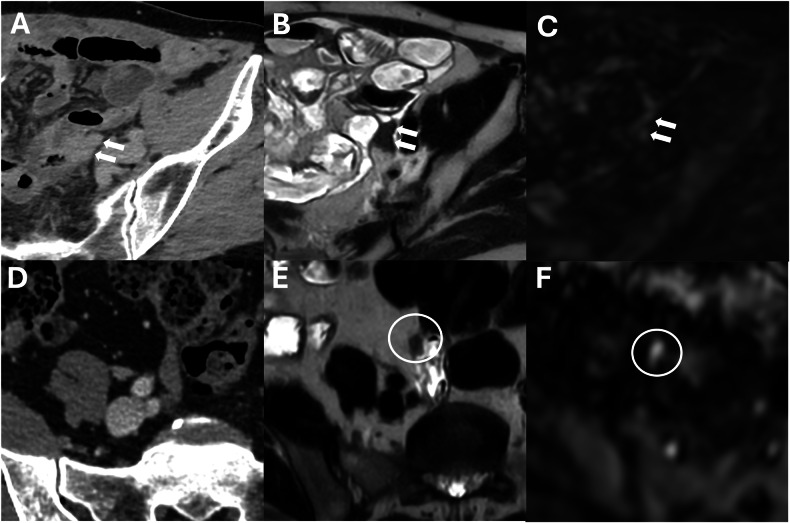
Ovarian cancer with peritoneal carcinomatosis in a 69-year-old woman (A–C). A CT delayed phase, axial plane showed a nodular implant in the left iliac fossa (arrows), confirmed in MRI T2w and DWI (b = 800 mm^2^/s) (arrows) (B, C). Colon adenocarcinoma with peritoneal carcinomatosis in a 64-year-old woman (D–F). MRI T2w (E) (circle) demonstrated a nodular implant in the distal ileum, confirmed in DWI (b = 800 mm^2^/s) (F) (circle), but not evident on CT portal-venous phase (D)
